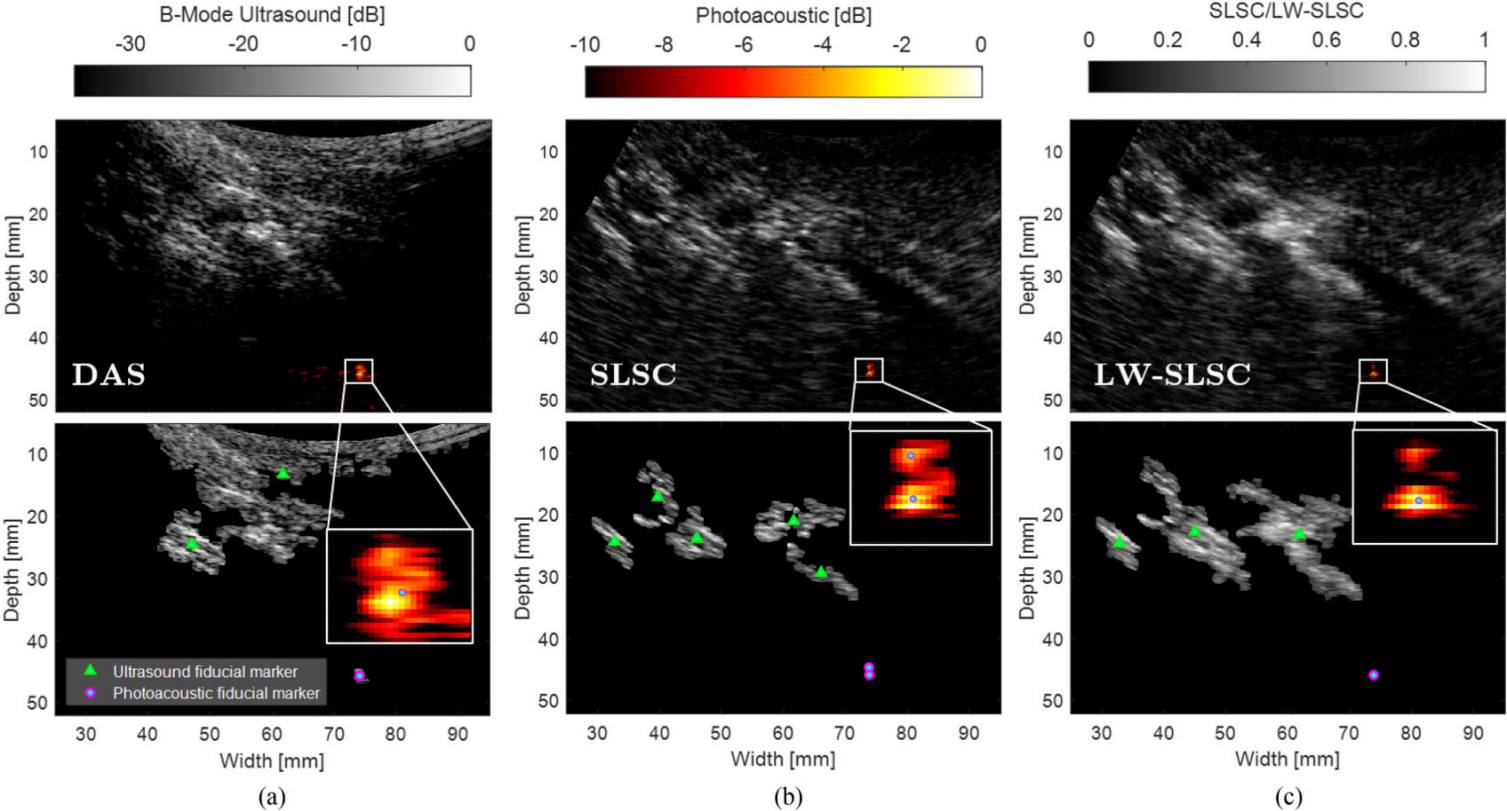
Examples of photoacoustic images overlaid on ultrasound images from an oblique view of L3-L5 vertebrae reconstructed with (a) DAS, (b) SLSC and (c) LW-SLSC ultrasound and photoacoustic beamforming. Top row: beamformed images. Bottom row: segmented masks. The triangles and circles represent the center of mass of isolated components from ultrasound and photoacoustic images, respectively, which are later combined and used as landmarks for CT registration. The insets show magnified views of the photoacoustic signal originating from the fiber tip.
